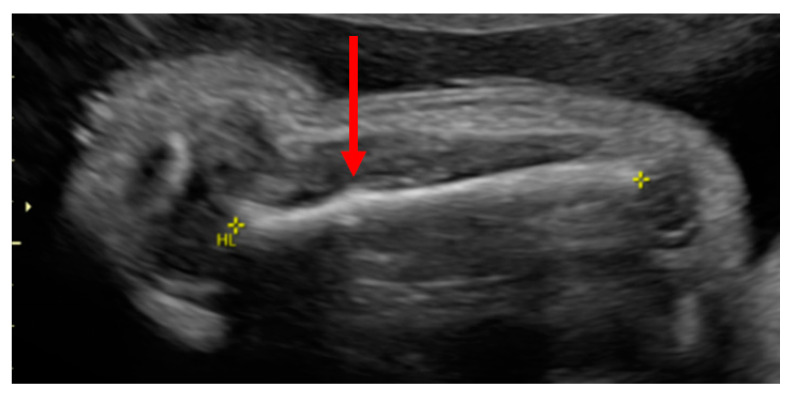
Prenatal ultrasound at 32w1d of gestation. The fetal survey showed a transverse linear fracture line through the proximal right humeral diaphysis with some associated bowing and callus formation. Holick MF, copyright 2021.
